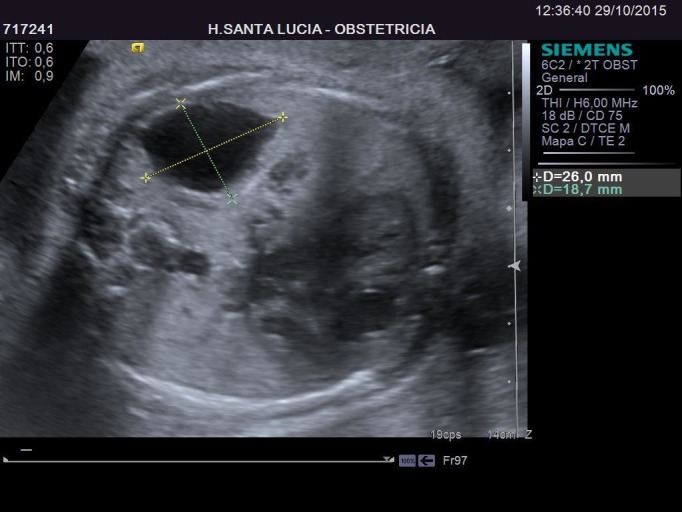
Ultrasound - Transversal View This figure shows the size of the congenital adenomatoid malformation.
